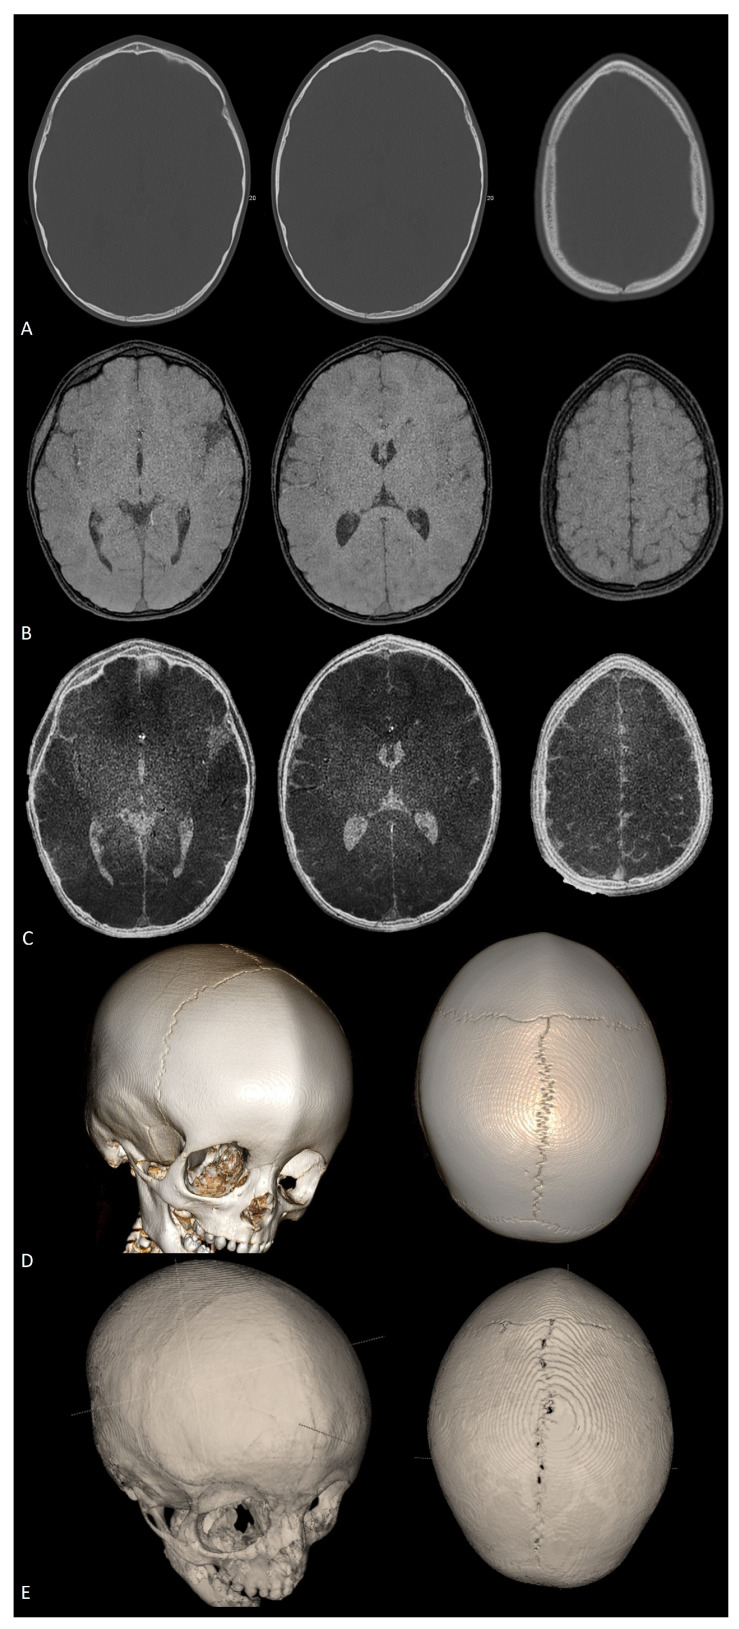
Axial CT scan (A), axial black-bone MRI (B), and axial black-bone MRI CT-like representation (C) at comparable levels in the same patient shown in Figure 11; 3D CT reconstruction (D) and 3D black-bone imaging postprocessing reconstruction (E) demonstrating comparable visualization of cranial sutures, metopic ridge, and overall skull deformity.
